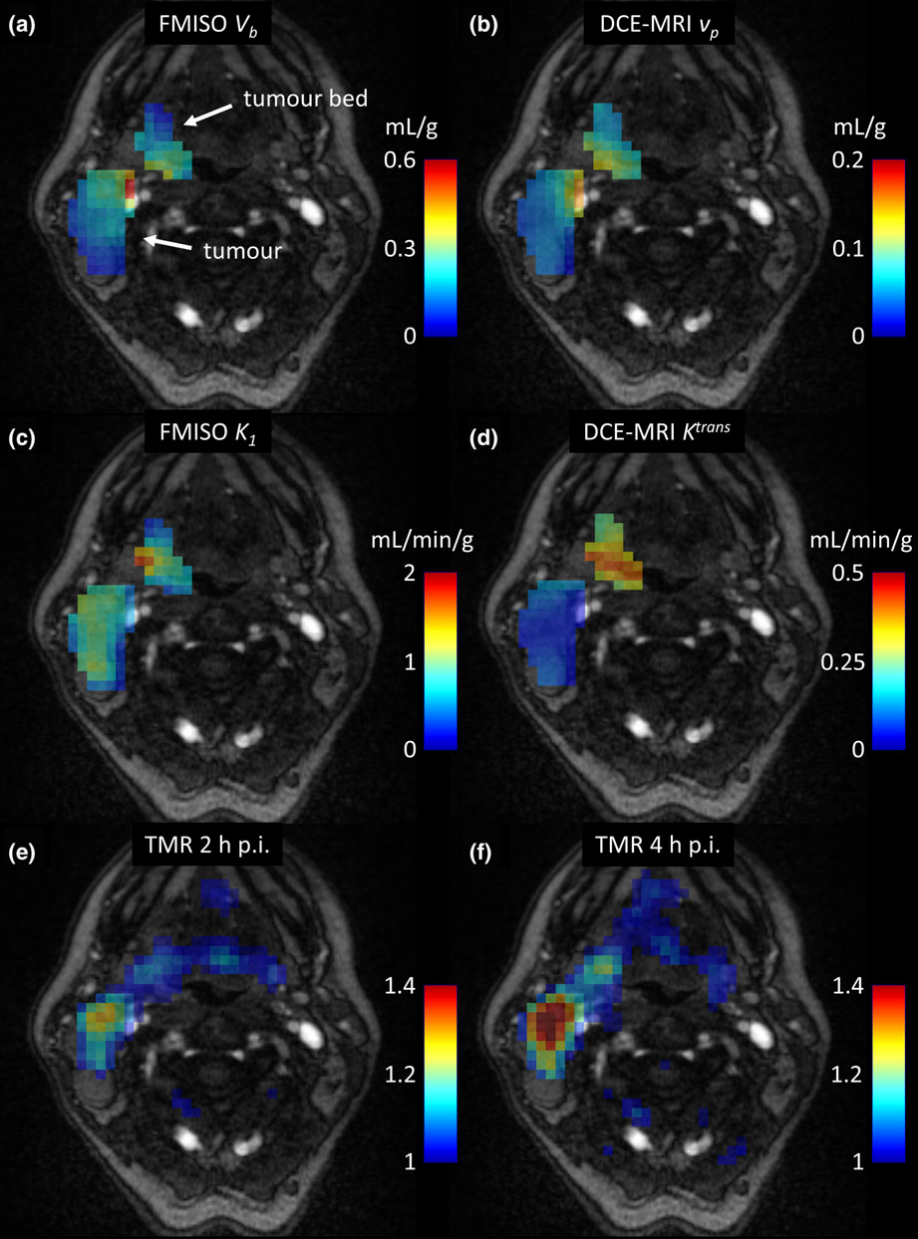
Contrast‐enhanced MRI images of the patient P2, overlaid with the FMISO
